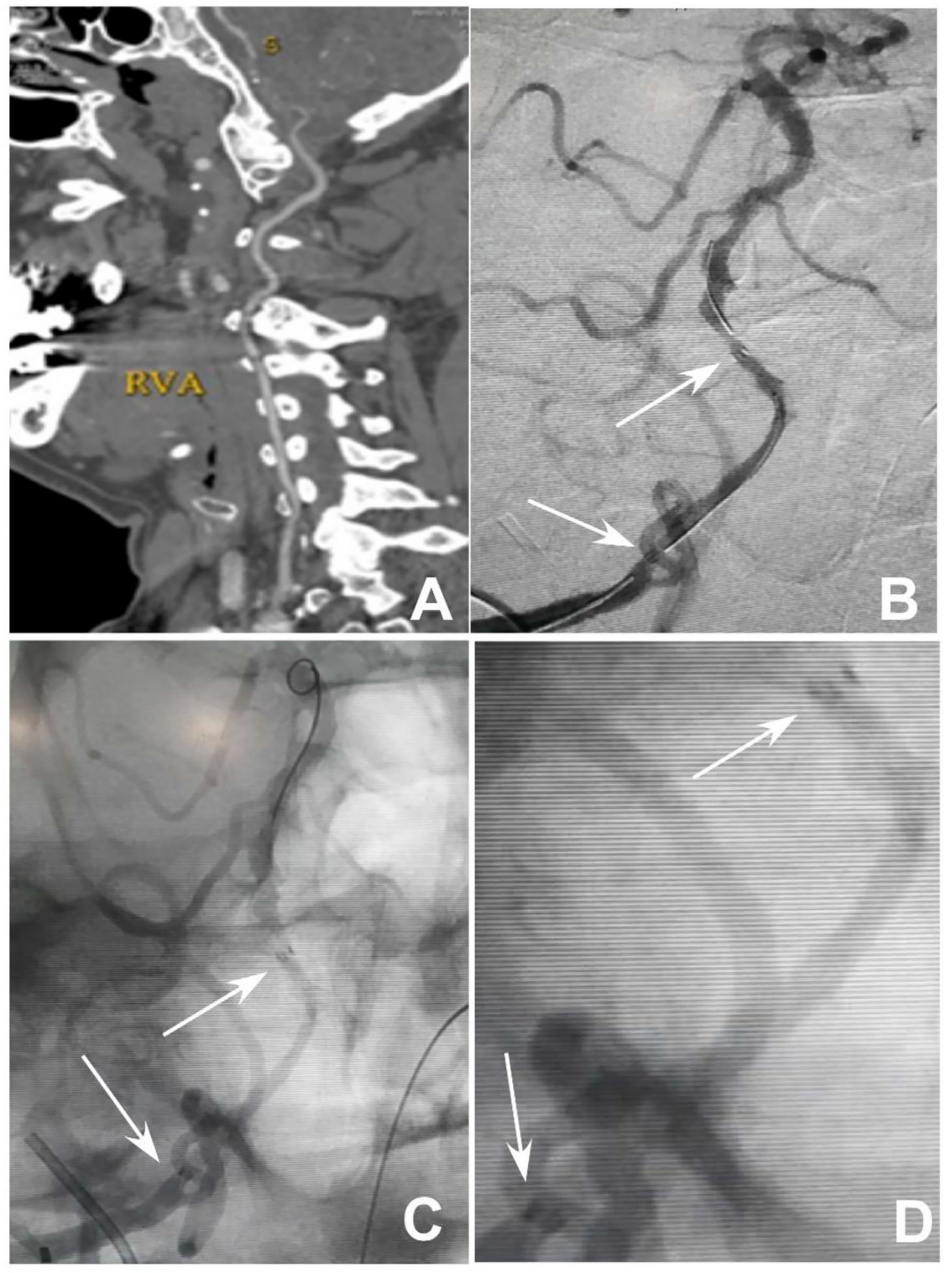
Endovascular treatment of V4 segment occlusion of the right vertebral artery. (A) Computed tomography angiography revealed occlusion of the V4 segment of the right vertebral artery without calcification of the occluded artery. (B) After balloon expansion of the occluded segment, an Enterprise stent was deployed at the occluded segment. (C,D) After deployment of the stent with the micro-guidewire still in the artery, the proximal and distal markers of the stent were spread out with patency of the stent. (D) is the local enlargement of (C) between the proximal and distal markers of the stent. Arrows indicate the proximal and distal markers of the stent.
